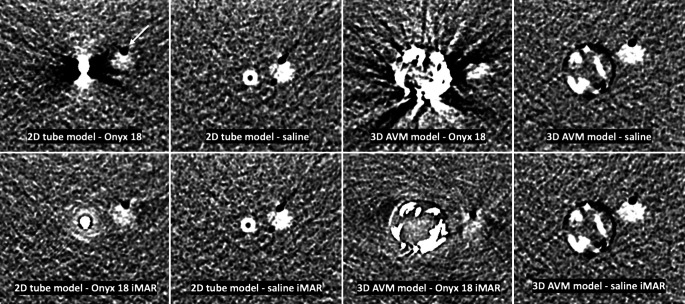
Representative CT images of the original and the post-processed images of both in vitro models with the experimental hemorrhage adjacent. CT images are presented in a standard brain window with a width of 80 HU and a level/center of 40 HU. Comparing the original (no iMAR) and the post-processed (with iMAR) images, a better definition of the experimental hemorrhage is possible after artifact reduction. Since the DMSO-compatible tubes still feature limited radiopacity, especially on the images of the 2D tube models, minor tube-associated artifacts can be observed. Note the small preparation artifact (*white arrow*) of the experimental hemorrhage
